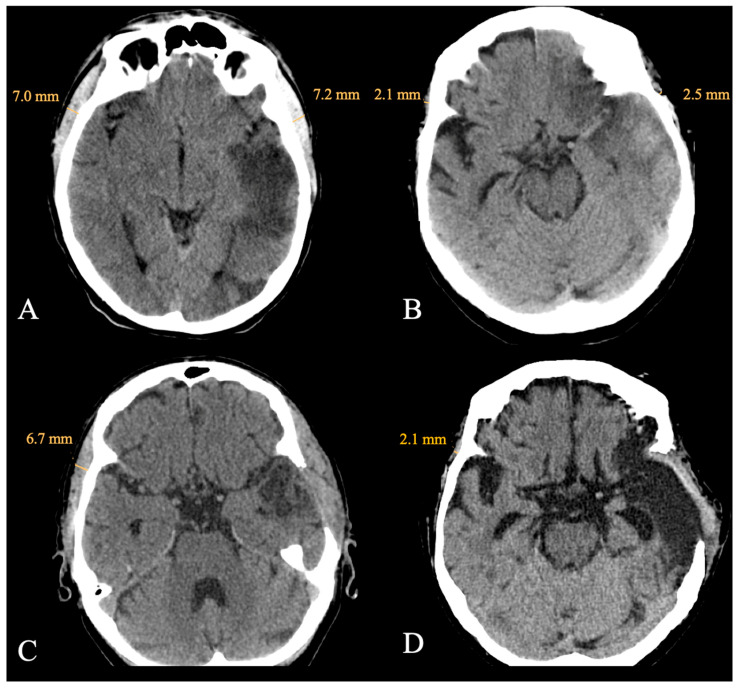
Representative axial cranial CT images demonstrating assessment of temporalis muscle thickness (TMT). (A) Patient with preserved TMT prior to decompressive craniectomy (mean bilateral TMT = 7.1 mm). (B) Patient with reduced TMT prior to decompressive craniectomy (mean bilateral TMT = 2.3 mm). (C) Same patient as in (A) after decompressive craniectomy, showing preserved contralateral TMT prior to cranioplasty (contralateral TMT = 6.7 mm). (D) Same patient as in (B) after decompressive craniectomy, demonstrating persistently reduced contralateral TMT prior to cranioplasty (contralateral TMT = 2.1 mm).
